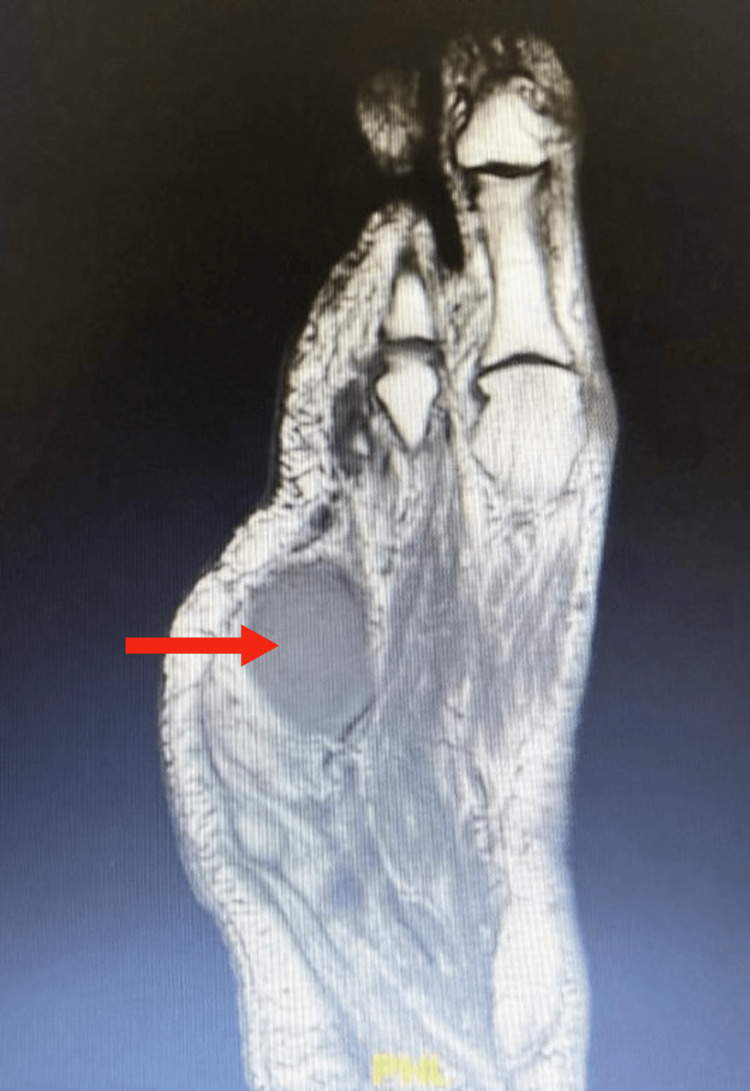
Sagittal T1-weighted MRI of the foot post amputation Large abscess with fluid collection underneath the fourth and fifth metatarsal amputation sites measuring 5.3 cm x 4 cm x 2.5 cm
